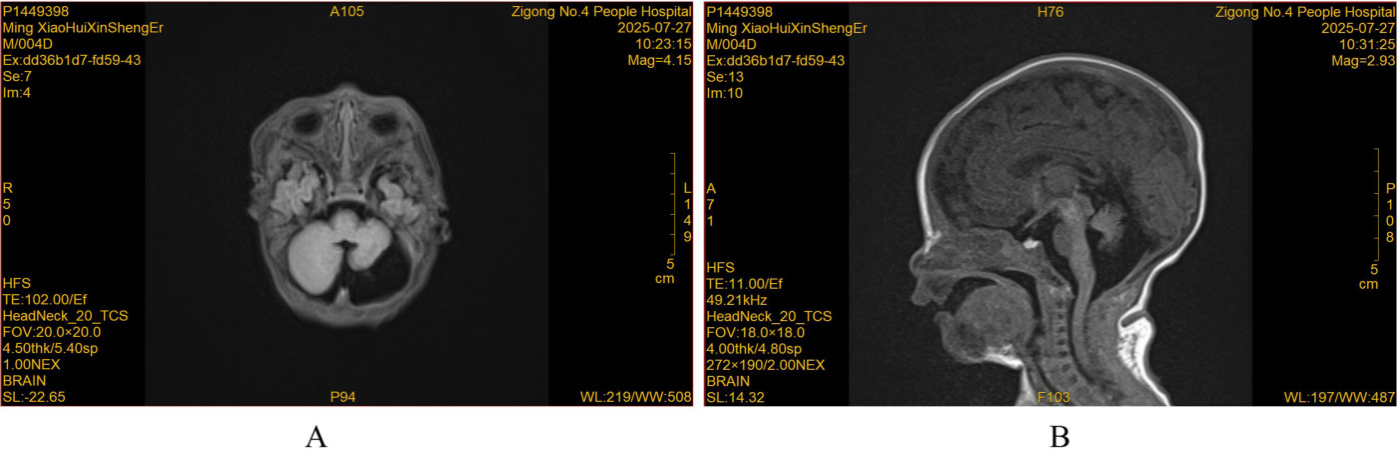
MRI of the brain. (A) Coronal scan (T2W1_blade dark fluid): complete absence of the cerebellar vermis and hypoplasia of the left cerebellar hemisphere. (B) Sagittal scan (T1W1_qtse dark fluid): posterior fossa cyst communicating with the dilated fourth ventricle.
